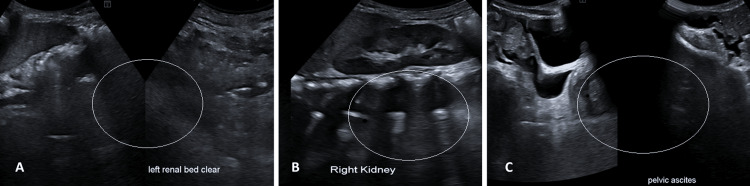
Ultrasonography (25-03-2025) showing no sonographic evidence of disease recurrence in the left nephrectomy bed (A), with a normal right kidney (B) and mild pelvic ascites (C).
